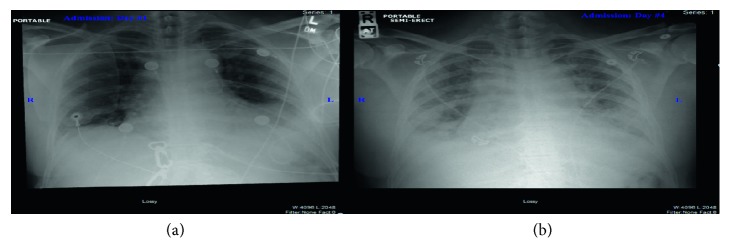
Portable chest radiography. (a) Defibrillator pad over the right chest wall. The lung is clear with sharp costophrenic angles bilaterally. Mild subsegmental atelectasis at the lung bases is seen. There are no airspace infiltrates, focal consolidation, pleural effusion, or pneumothorax. (b) Enlarged cardiomediastinal silhouette. Interval development of small bilateral pleural effusions and pulmonary congestion occurs.
